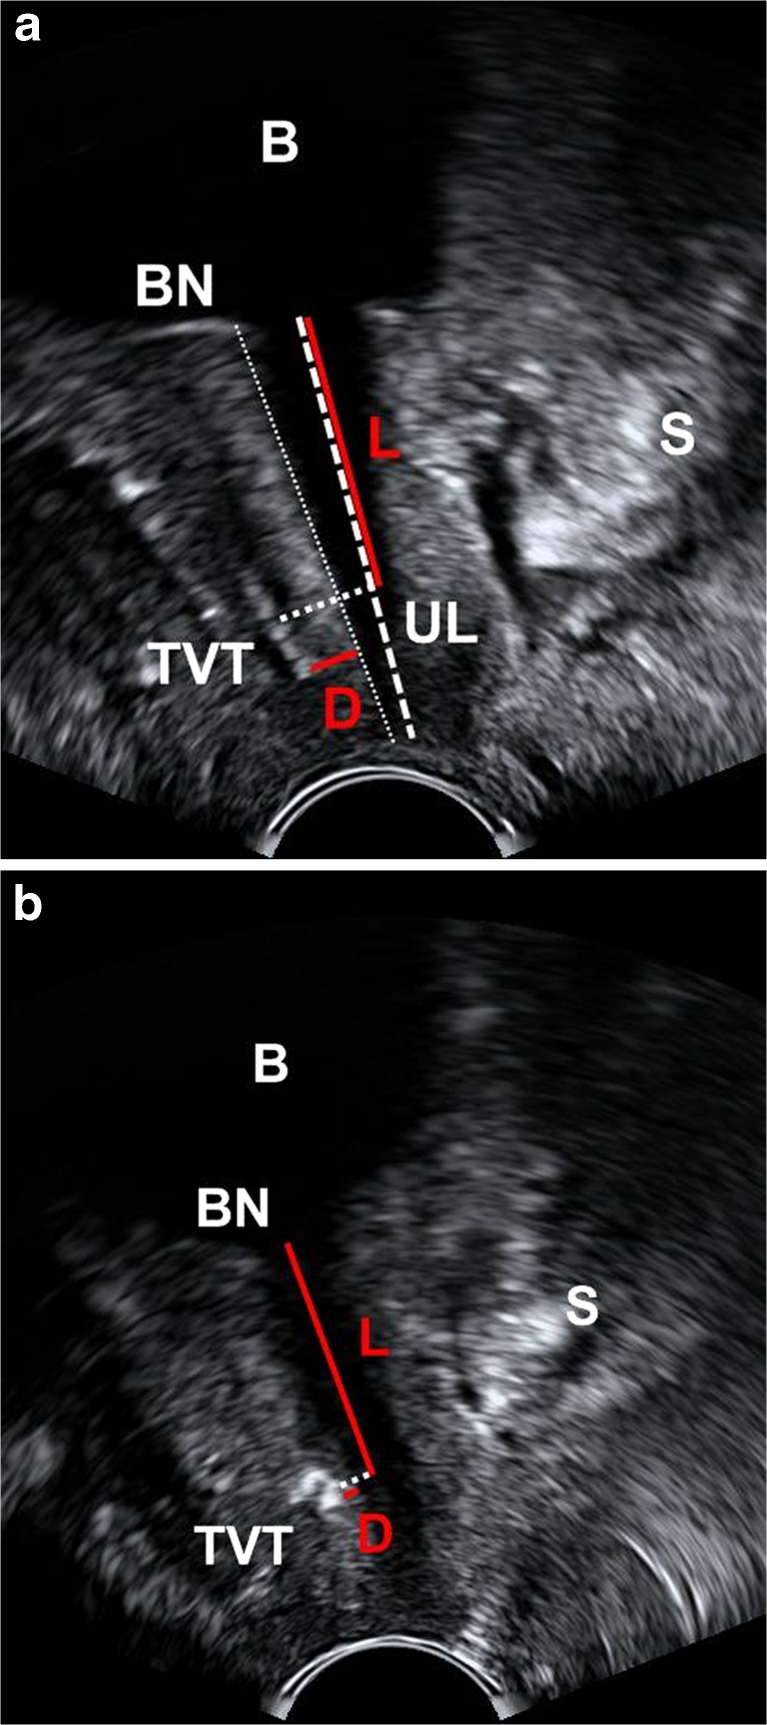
Ultrasound localization of the sling at rest for diagnosis of a dystopic sling or dysfunction. a Pelvic floor ultrasound image showing the optimal position of the tension-free vaginal tape (*TVT*) b Pelvic floor ultrasound image showing a TVT positioned too close to the urethra with excessive tension (sling curling). *B* bladder, *BN* bladder neck, *S* symphysis; *D* shortest distance between the sling and the longitudinal smooth muscle (LSM) complex; *UL* length of the entire urethra (bladder neck to urethral papilla) as measured on ultrasound; *L* proportion of the urethra (bladder neck to middle of the sling) used to determine TVT position along the urethra (%)
